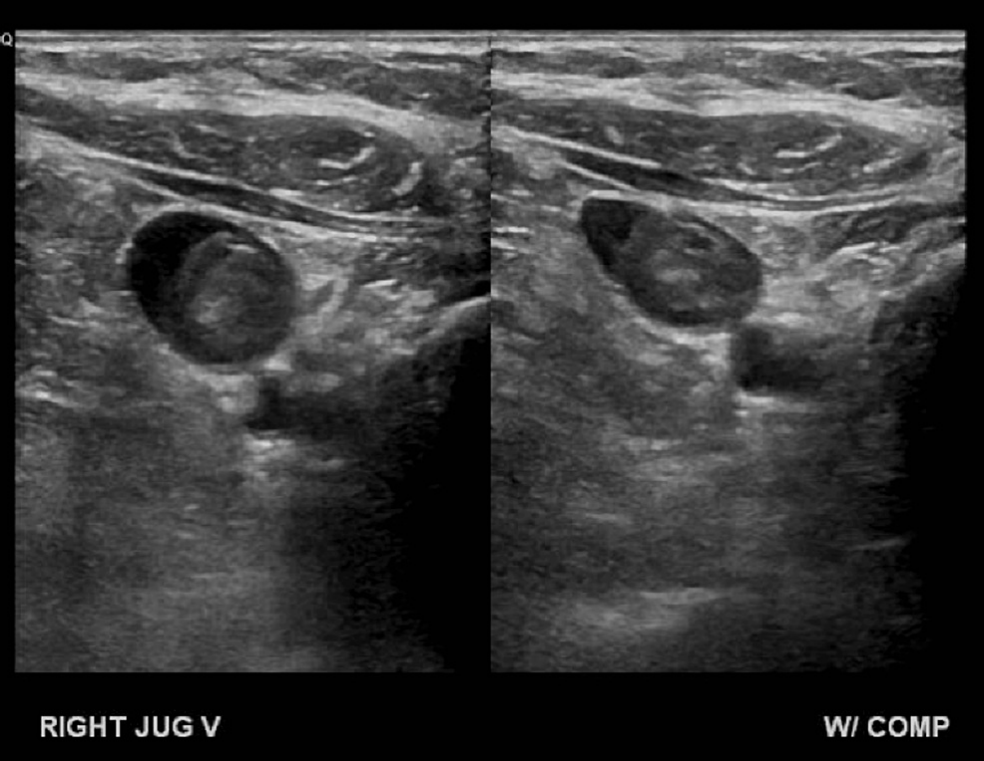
Duplex ultrasound of the right upper extremity shows right jugular vein thrombosis.
